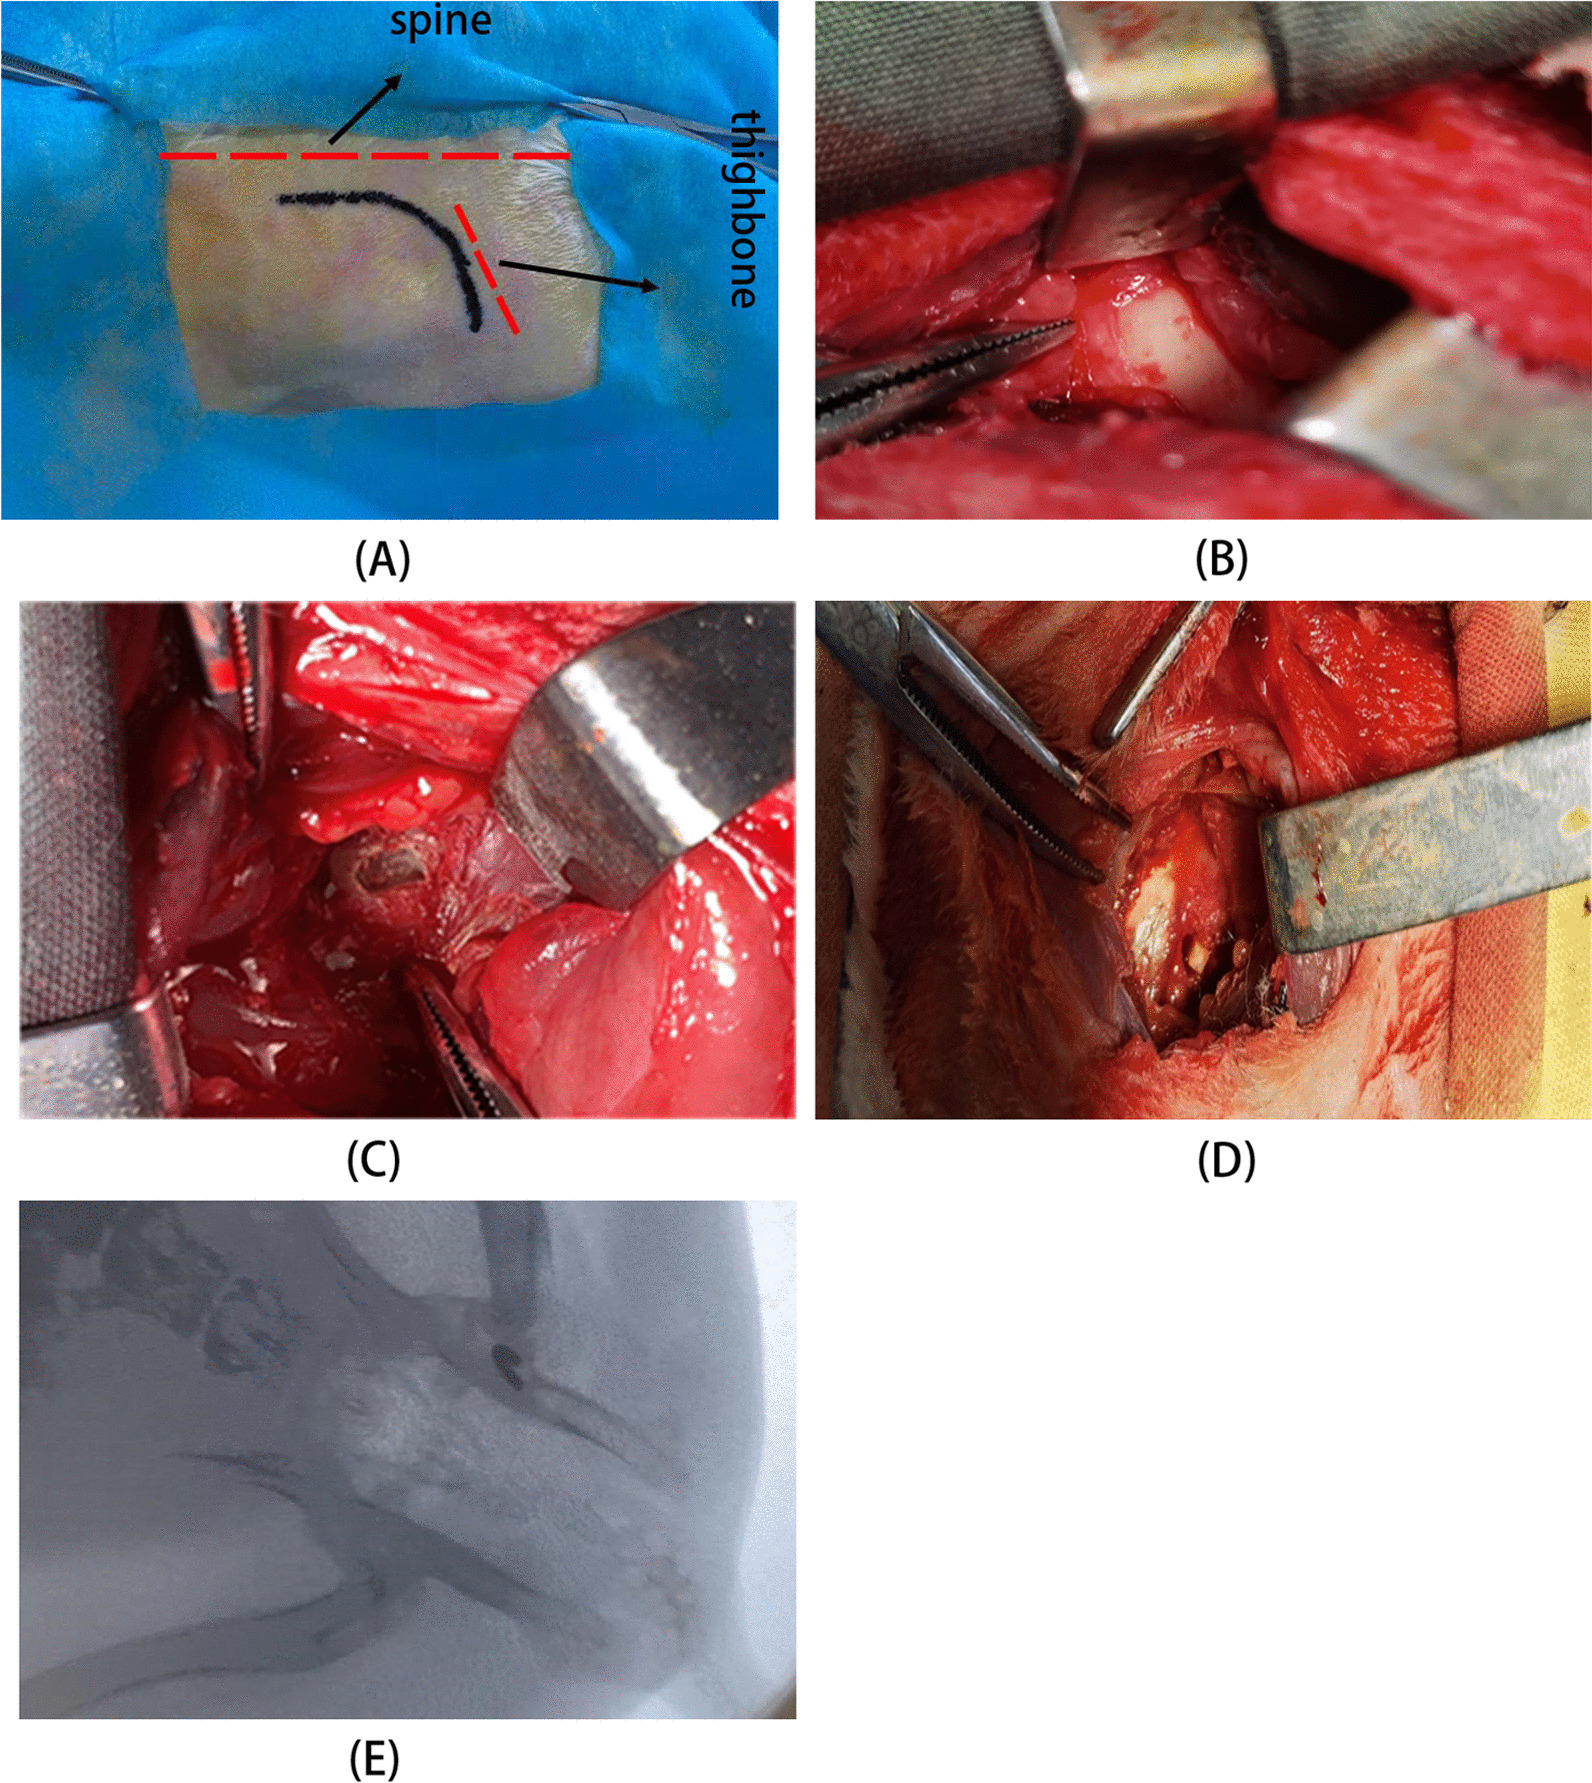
Operational procedures for creating the femoral head necrosis model. A Selection of the iliac crest and greater trochanter as the sites for an arched incision; B Sequential dissection of muscle and other soft tissues to expose the acetabulum; C Create a window approximately 3 mm away from the acetabular rim; D Implantation of the femoral head compression device. E Intraoperative X-ray imaging confirms the accurate placement of the bone window
